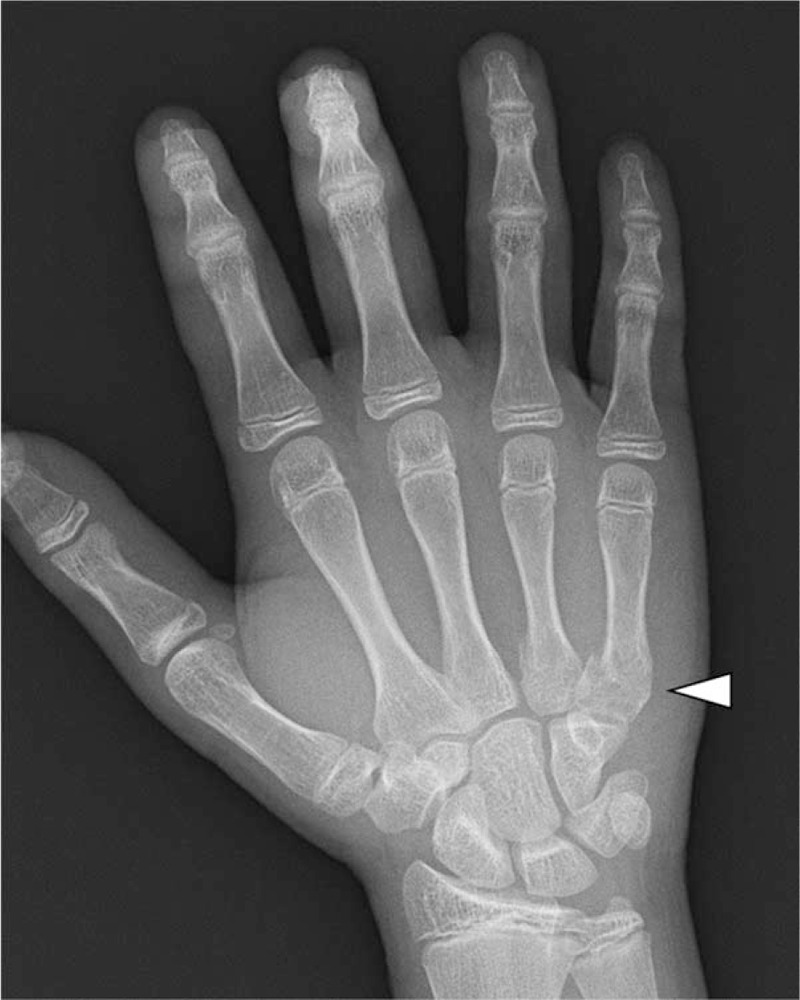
Pre-hospital X-ray: a right 4th and 5th metacarpal fracture called a boxer's fracture (white arrowhead) was noted.
